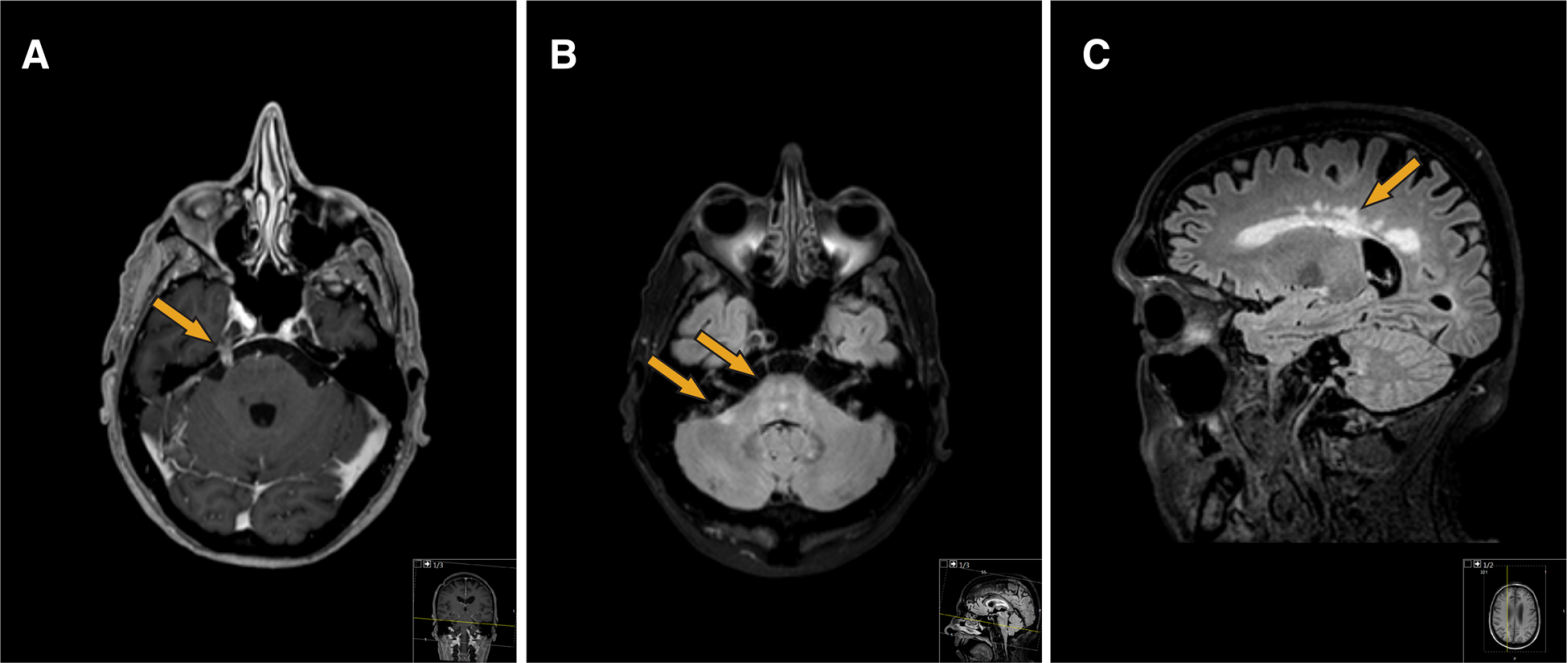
a 3D heavily T1-weighted gradient-echo pulse-sequence obtained after administration of intravenous gadolinium shows pathological contrast enhancement of the right trigeminal nerve, in the cisternal segment. b This 3D FLAIR turbo spin-echo pulse-sequence reveals hyper intense signal abnormalities in in the pons and right middle cerebellar peduncle. c Paramedian sagittal FLAIR sequence displaying periventricular confluent hyperintense signal abnormalities most certainly related to inflammation due to neuroborreliosis
